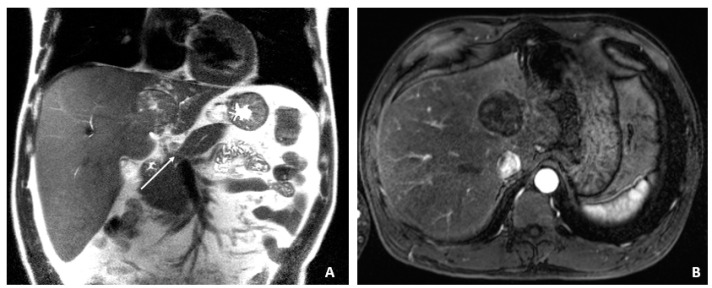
MRI at the time of diagnosis of HCC (December 2009). (A) The coronal T2-weighted image shows a mildly heterogeneous mass in the left hepatic lobe (arrow), corresponding to hepatocellular carcinoma. (B) The axial T1-weighted fat-suppressed arterial-phase postcontrast image shows faint foci of hyperenhancement within the lesion, characteristic of HCC.
